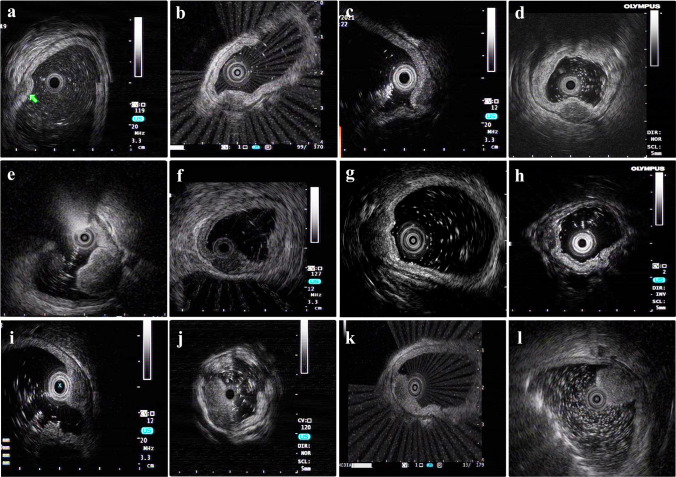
Endoscopic ultrasound images of patients who underwent R1 resection. Note: a–j Boundary between the submucosa and muscularis propria at the lesion is not clear; k–l boundary between the submucosa and muscularis propria is clear; R1 resection, incomplete resection
